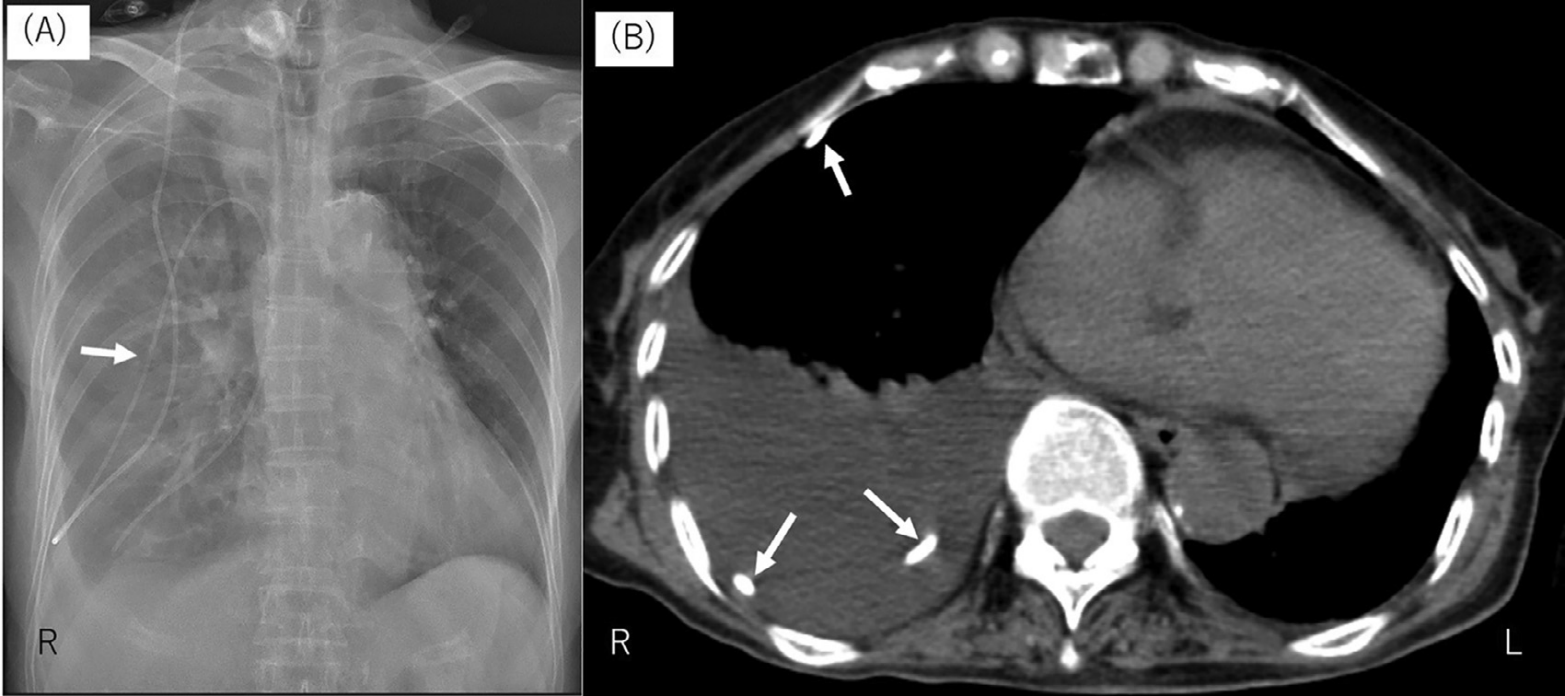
Chest radiograph (A) and computed tomography scan (B) taken on second admission to our hospital, on day 156 after the first ventriculoperitoneal shunt procedure, showing massive pleural effusion and abnormal position of the distal catheter (white arrows) in the right lung field.
